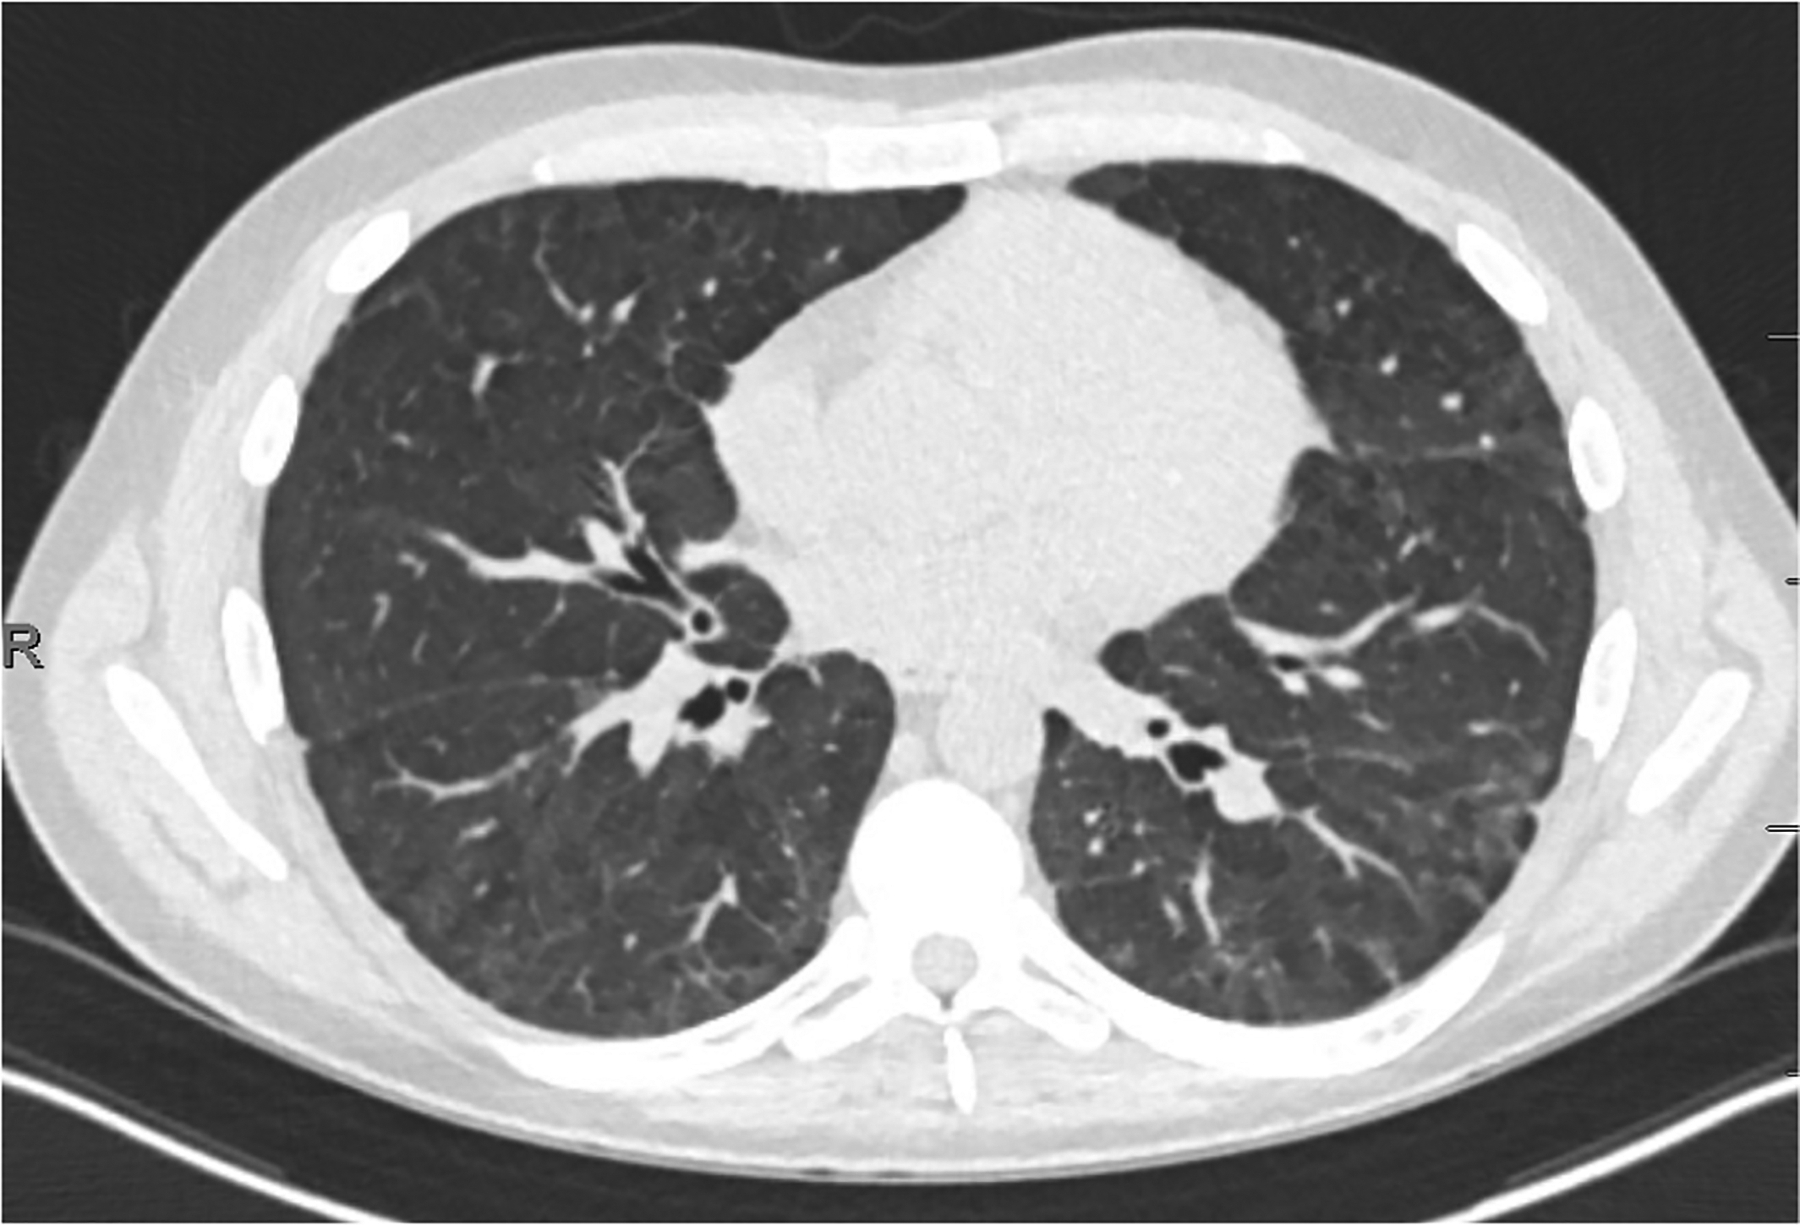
Axial CT image corresponding to 30 days post discharge and one week post completion of pirfenidone therapy. Continued improvement in centrilobular ground glass opacities with interval resolution of consolidative components and improving interlobular septal thickening. Evolving subpleural linear and bandlike opacities, greatest in the lingula and posterior lung bases.
